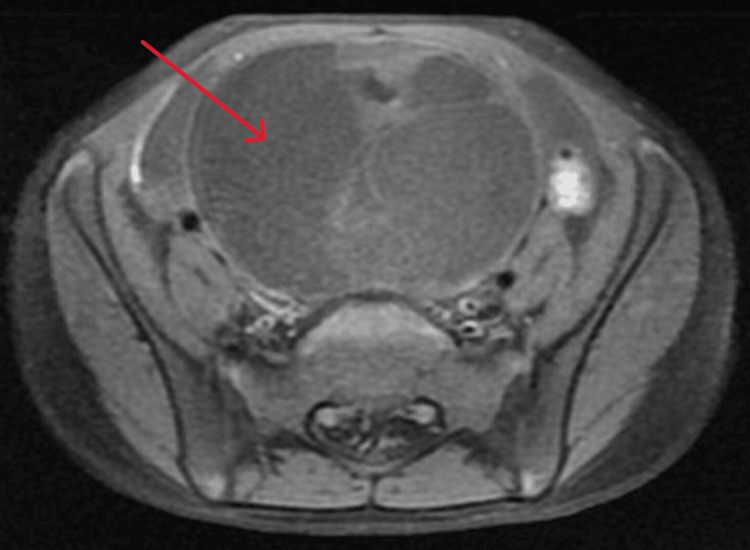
MRI findings indicating a large midline pelvic complex cystic lesion containing fat and calcification, most likely a mature cystic teratoma arising from the right ovary (arrow) The left ovary shows follicles, likely due to hormonal effects. There are no abnormalities noted in the bones or other significant findings.
